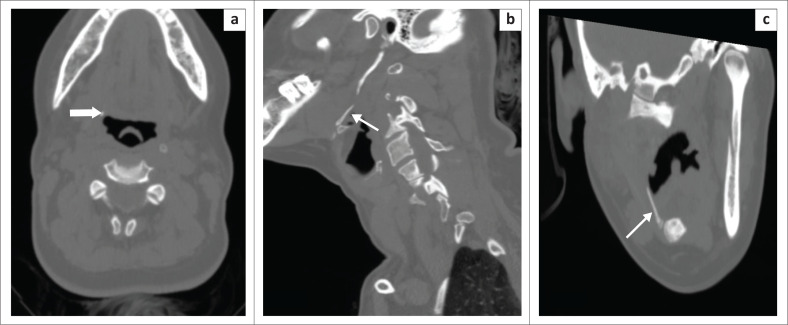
(a–c) Computed tomography images of a 65-year-old woman with a foreign body sensation in the right neck following a meal: (a) Axial image shows suspicious hyperdense material near the base of the tongue (arrow) on the right side, mimicking a foreign body. However, (b) sagittal reformatted and (c) 3D multiplanar reformation (MPR) images confirmed it to be the greater cornu or horn of the hyoid bone.
